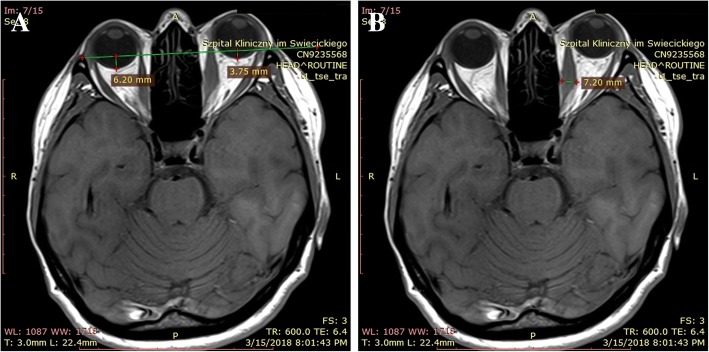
a. Left-sided exophthalmos demonstrated by MRI in an axial plane. b. Thickening of the left medial rectus muscle demonstrated by MRI in a transverse plane
